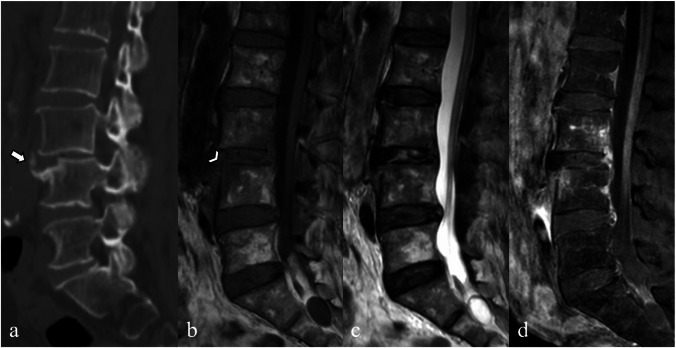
A sagittal computed tomography image (a) of the lumbosacral spine of a 70-year-old female patient with brucellar spondylitis. Bone erosion is observed at the anterosuperior corner of the L4 vertebral body, termed the “Pedro Pons’ sign,” along with reactive bone sclerosis with anterior osteophytes that form a “parrot’s beak” appearance (arrow). Sagittal T1-weighted (b), T2-weighted (c), and fat-suppressed contrast-enhanced T1-weighted (d) images show focal bone destruction at the anterosuperior endplate of L4 vertebral body (arrowhead in image b) with enhancement of the opposing endplate of L3–L4 vertebral bodies and mild hyperintense T2-weighted signal changes of the intervertebral disc
